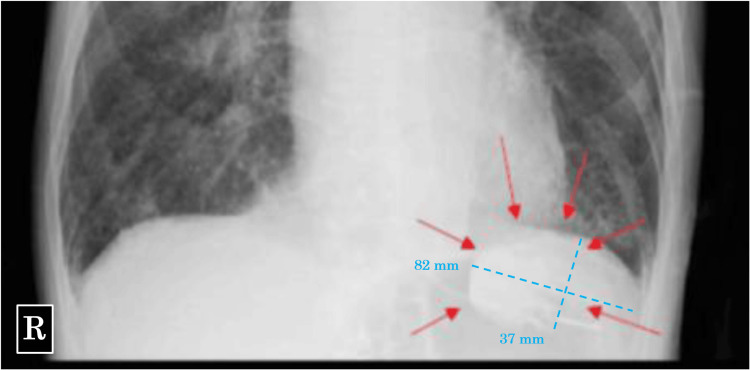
Chest X-ray on day 3 Chest X-ray shows radiopaque material (red arrow) in the stomach.
